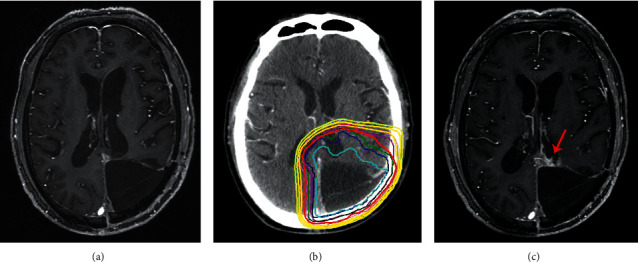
Example of nSVZE. A 66-year-old male with pathologically confirmed anaplastic astrocytoma in the left parieto-occipital lobe. His OS was 510 days. Axial contrast-enhanced T1-weighted image 72 h after resection (a). Axial contrast-enhanced CT image with treatment-planning overlay shows the volumes treated with 60 Gy (blue outline) and 50 Gy (red outline) (b). There was nSVZE lesion at the left trigonum in follow-up MRI 6 months after completion of radiotherapy (c). nSVZE, new subventricular zone enhancement.
